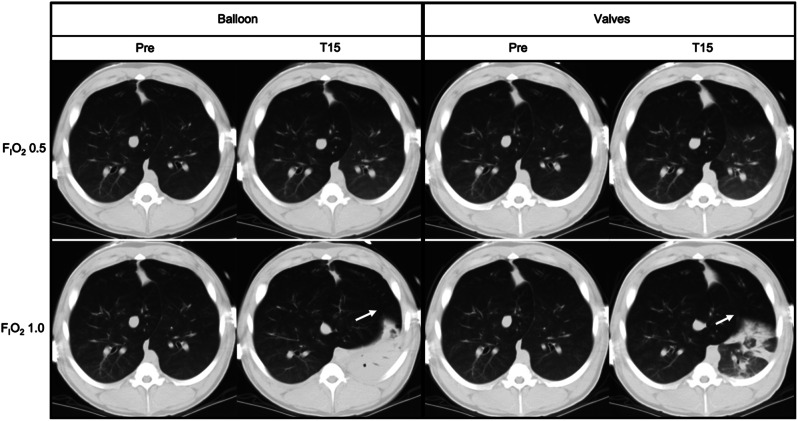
Computed tomography images. Computed tomography (CT) images from both occlusion methods and fraction of inspired oxygen (F_I_O_2_). Time points were *Pre* (under the *standard mechanical ventilation settings*) and *T15* (15-minute occlusion). The *Pre* vs. *T15* CT images were unequivocally different with F_I_O_2_ 1.0. In addition, the *T15* CT images with F_I_O_2_ 1.0 were also clearly different between the two occlusion methods. The F_I_O_2_ 1.0 resulted in complete atelectasis with the balloon and partial atelectasis with the valves. The images at *T15* with F_I_O_2_ 1.0 also show a visible expansion of the accessory lobe displaced towards the left hemithorax (white arrow), which was more prominent in the *Balloon Occlusion Method*
